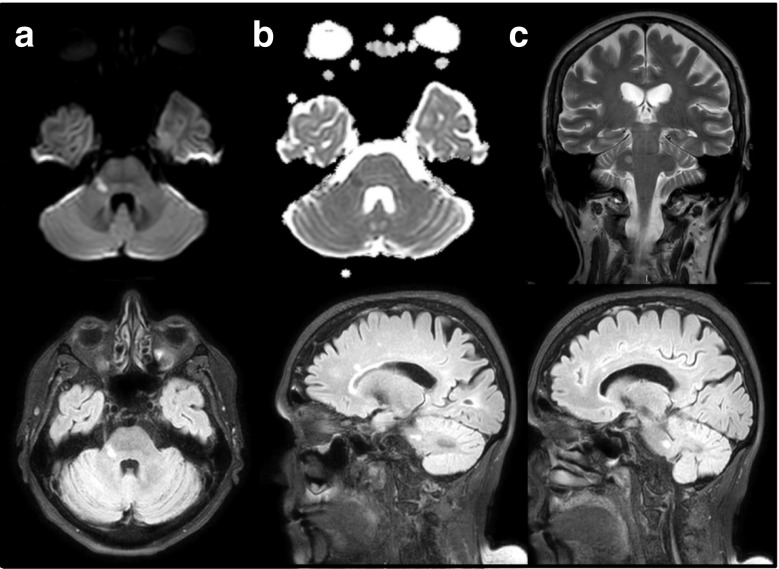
Ischemic stroke of the right brachium pontis, corresponding to the trigeminal root entry zone. a-b show high signal on DWI sequence which is confirmed by ADC maps to represent abnormal restricted diffusion. FLAIR sequence (c to f) display marked hyperintensity at the right trigeminal nucleus and sensitive root
